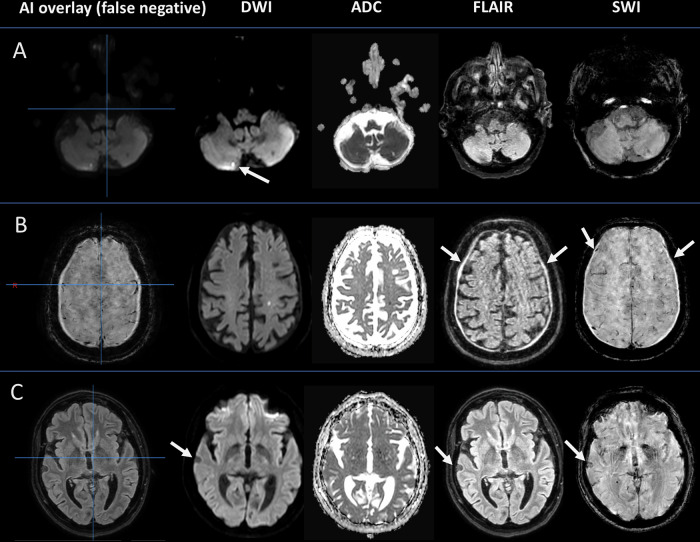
False negative predictions from AI tool in scans with motion artifacts. The left column displays the AI interface, and the right columns display sequences from PACS (DWI, ADC, FLAIR, and SWI). A 97-year-old male with decreased strength in the left upper extremity, facial palsy, and aphasia. Stroke MRI reveals a tiny acute ischemic lesion in the right cerebellum, overlooked by the AI tool. B 83-year-old male with right-sided facial palsy and right-sided hemiparesis. Stroke MRI shows bilateral hygromas/subdural hematomas, which are overlooked by the AI tool. C 66-year-old female with aphasia. Stroke MRI shows a tiny right-sided extra-axial tumor, not detected by the AI tool
